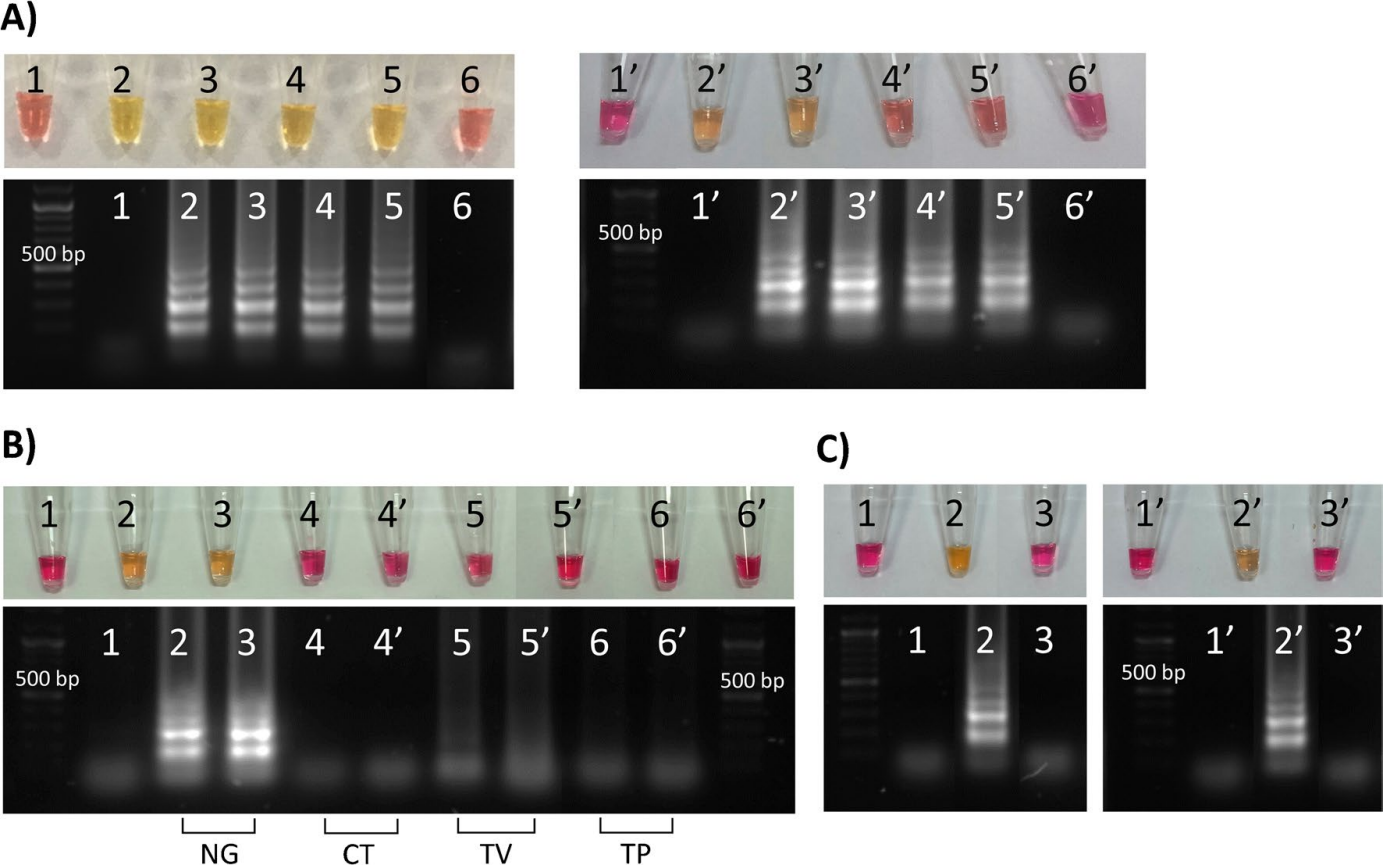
Investigation of tube-based colorimetric LAMP assay for detection of *N. gonorrhoeae* (NG) DNA. A Sensitivity of LAMP assay, two replicates of: 1, 1′ = no template control; 2, 2′ = 5 × 10^4^ NG copies; 3, 3′ = 5 × 10^3^ NG copies; 4, 4′ = 500 NG copies; 5, 5′ = 50 NG copies; 6, 6′ = 5 NG copies. Tubes were incubated at 65°C for 35 min. B Specificity testing of primers targeting porA pseudogene of NG with single DNAs: 1 = no template control; 2 = 5 × 10^3^ NG copies; 3 = 500 NG copies; 4, 4′ = 1.12 ng *C. trachomatis* (CT) DNA; 5, 5′ = 5 × 10^4^ copies *T. vaginalis* (TV) DNA; 6, 6′ = 4 × 10^4^ copies *T. pallidum* (TP) DNA. Tubes were incubated at 65°C for 30 min (2–3), 40 min (4, 4′) or 50 min (1, 5–6′). C Specificity testing of primers targeting porA pseudogene of NG with mixture of DNAs, two replicates of: 1, 1′ = no template control; 2, 2′ = 500 NG copies + 112 pg CT + 5 × 10^3^ TV copies + 4 × 10^3^ TP copies; 3, 3′ = 1.12 ng CT + 5 × 10^4^ TV copies; 4 × 10^4^ TP copies. Tubes were incubated at 65°C for 40 min
